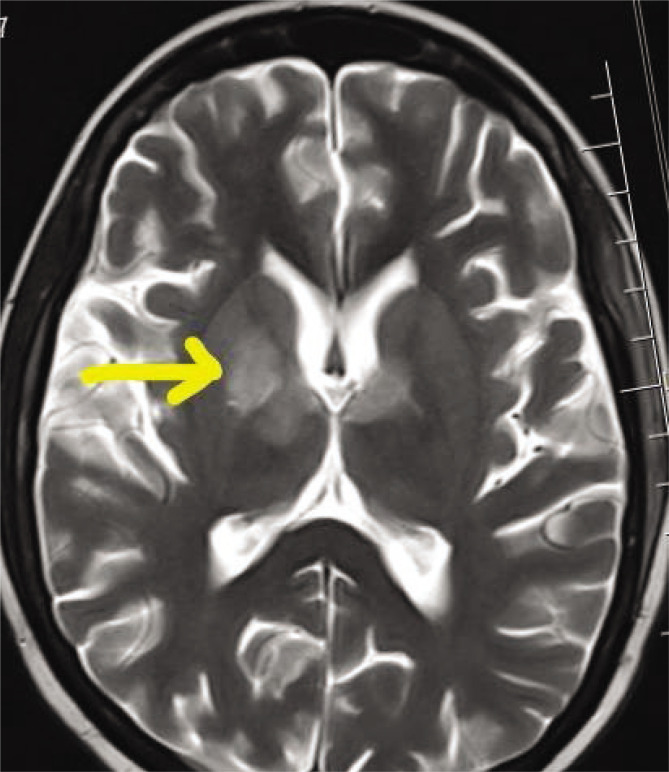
Axial T2 weighted images and FLAIR showing ill-defined hyperintense lesions in bilateral basal ganglia, thalami, and internal capsule.
